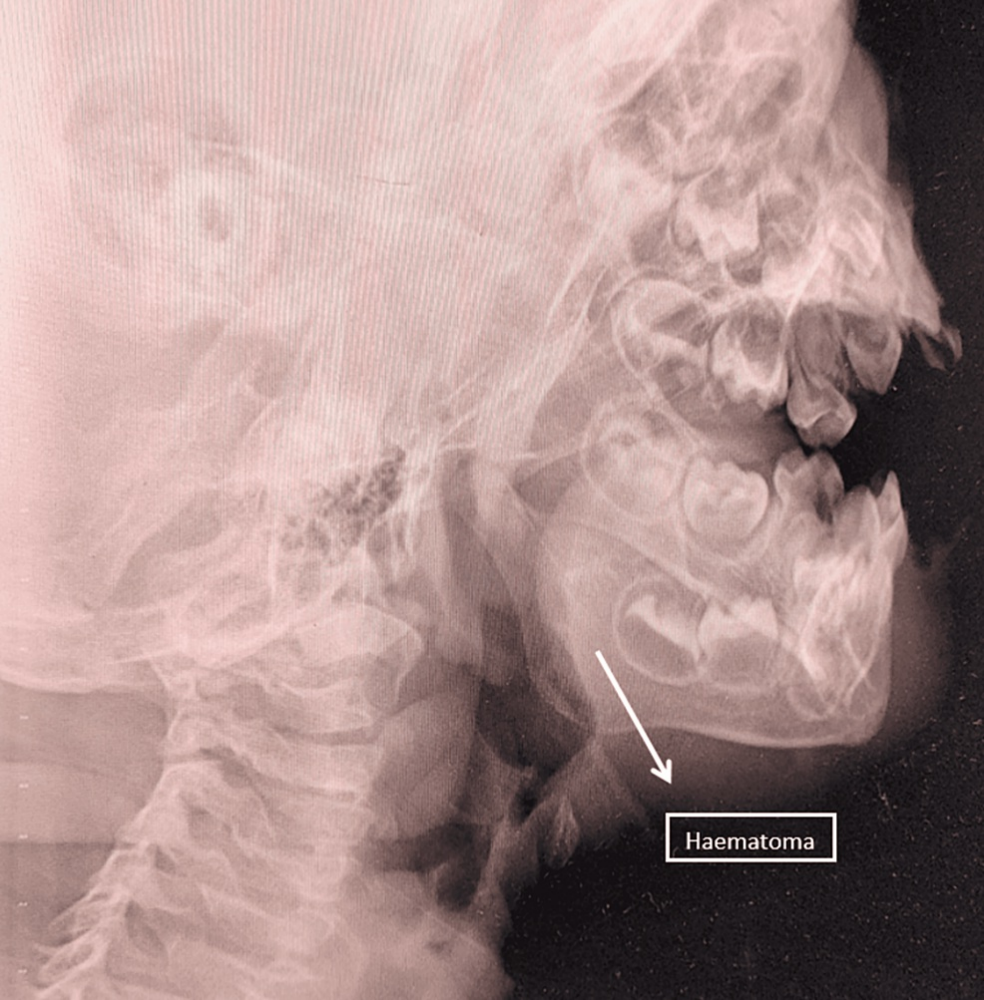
Lateral view of the neck and face X-ray reveals a haematoma
